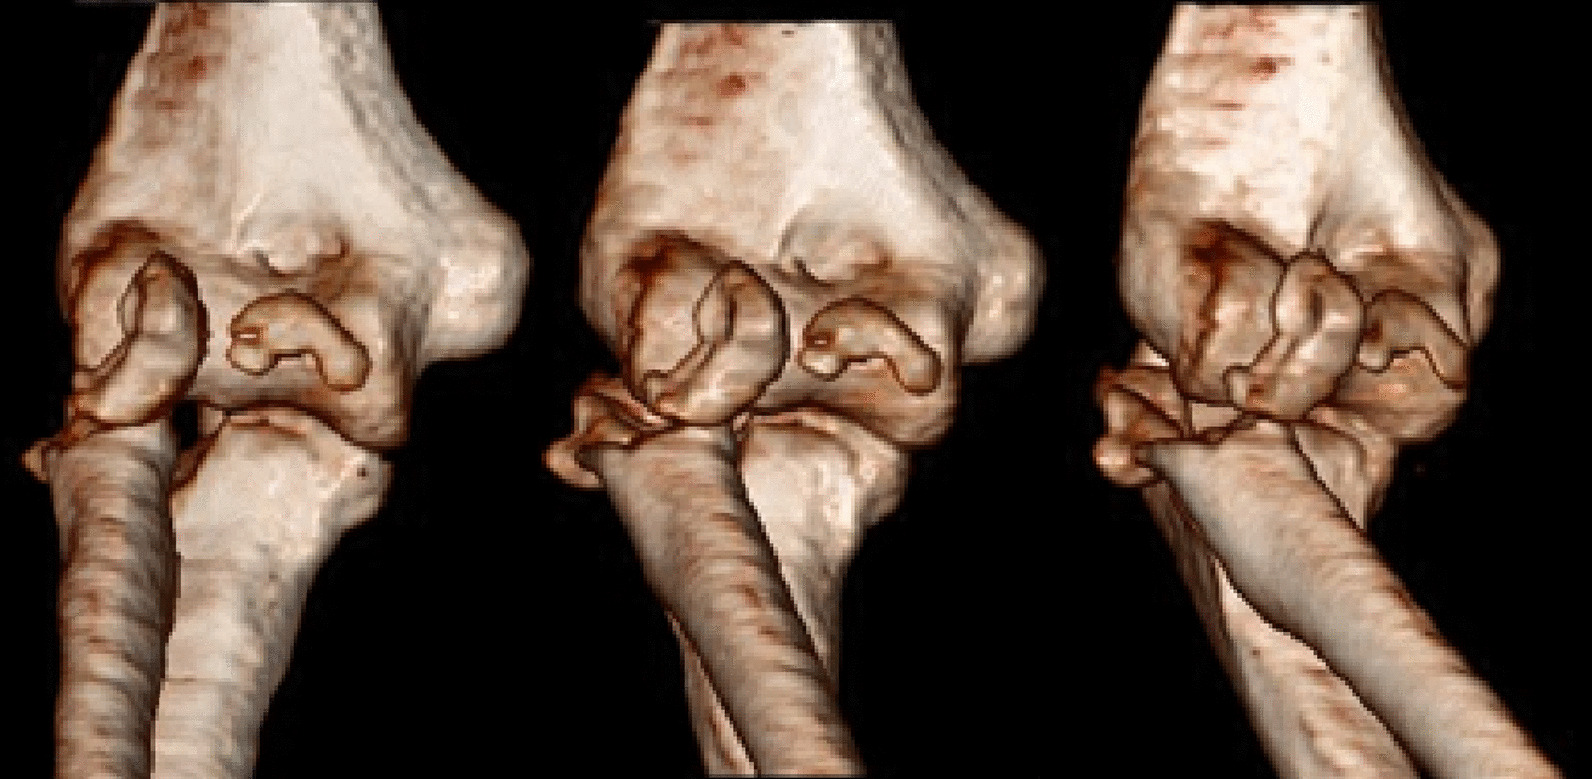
Right elbow computed tomography (CT) imaging; radial head fracture (Mason type 4) and coronoid fracture (Regan–Morris type 2) are described in great depth
